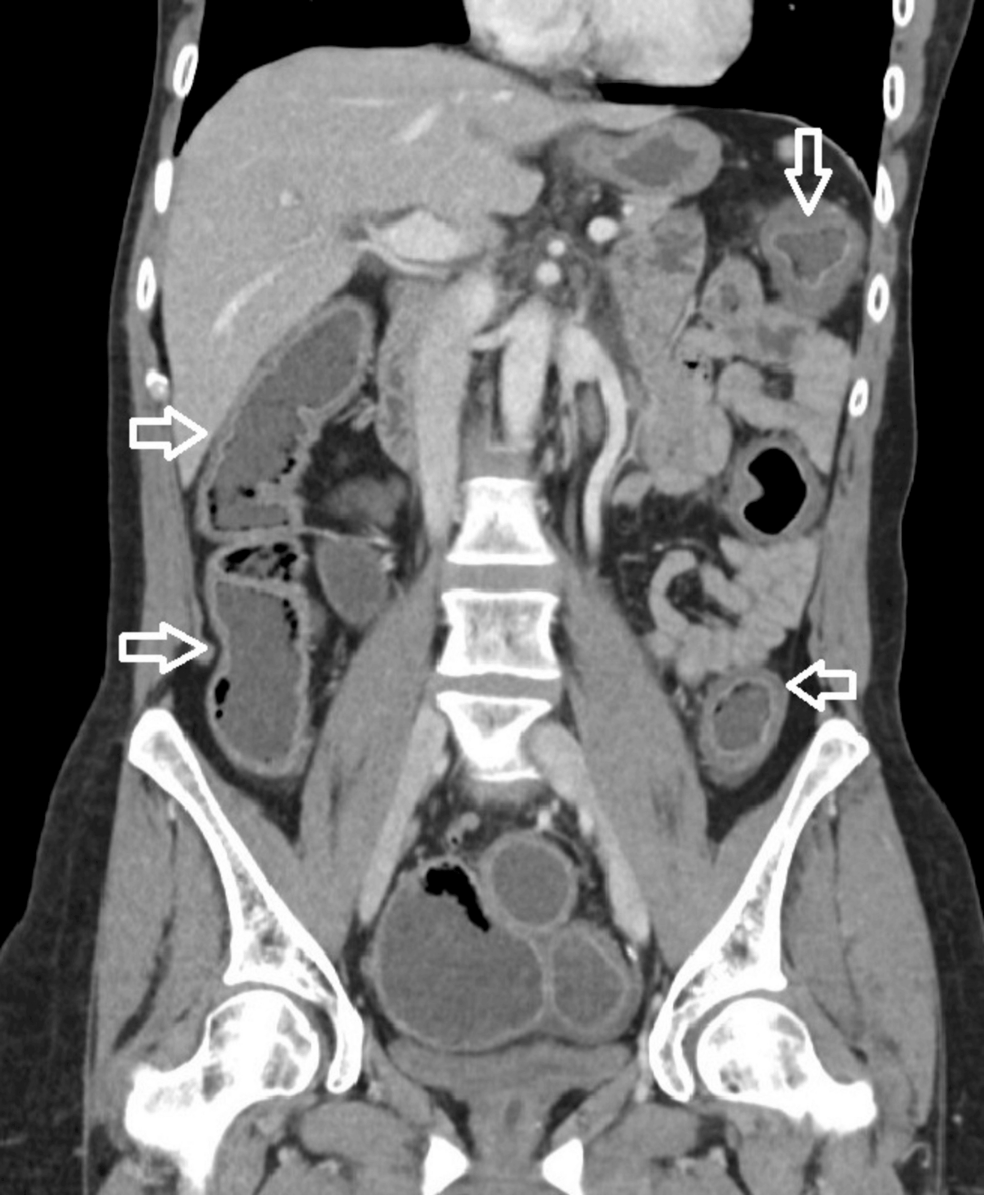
Coronal view of abdominopelvic CT Scan with contrast-the four white arrows point to diffuse colonic wall thickening and enhancement related to pancolitis, without evidence of bowel perforation or obstruction CT: computed tomography
